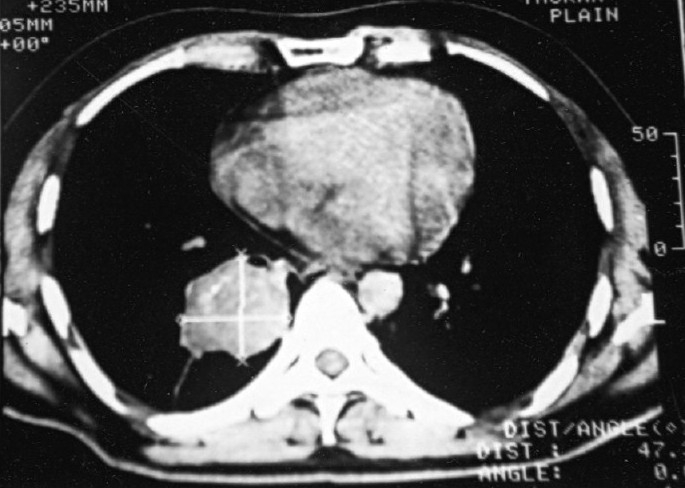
CT scan thorax showing soft-tissue density mass (carcinoid tumor) at medial segment of the right lower lobe
